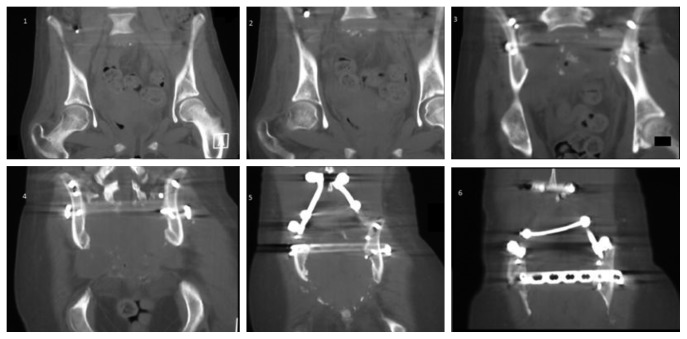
Postoperative CT (coronal slices) showing a complete removal of the sacrum.
